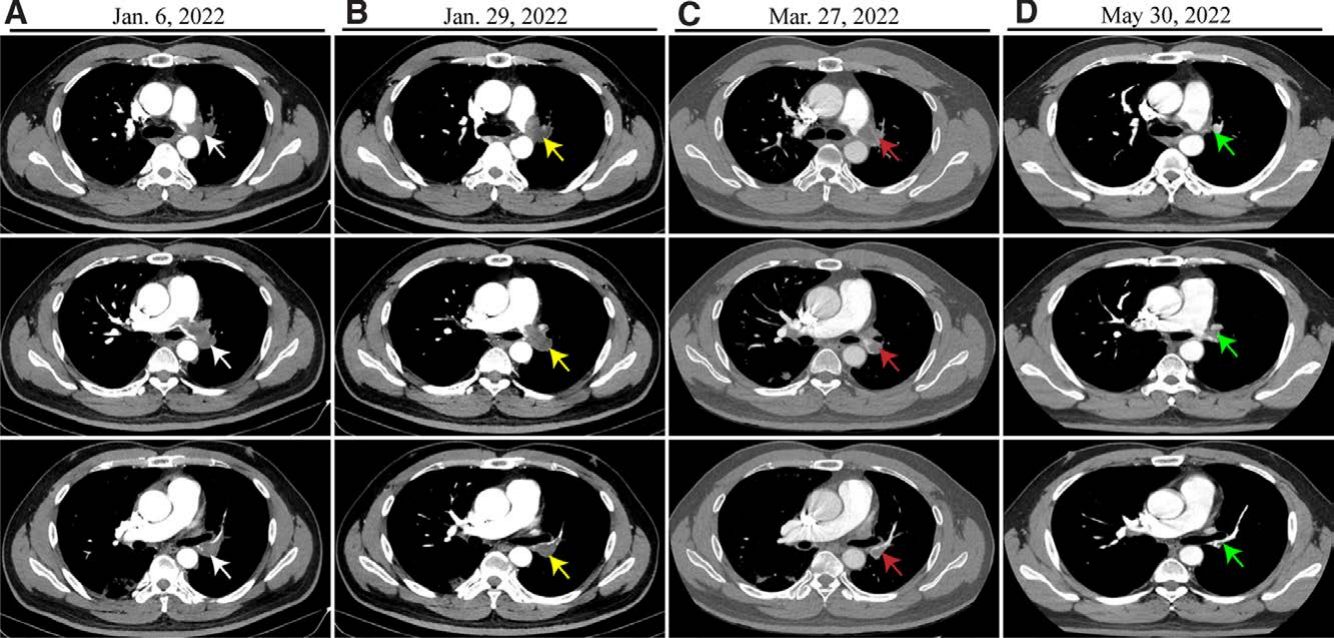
Thoracic CTPA scan images before and after therapy. (a) CTPA scan on admission (white arrow). CTPA images indicated that the endovascular lesions reduced gradually before the second (b) (yellow arrow), the third (c) (red yellow) chemotherapy and after the fifth (d) (green arrow) chemotherapy. Three images represent 3 different frames from the upper to the down. CTPA = Computed tomography pulmonary angiography.
